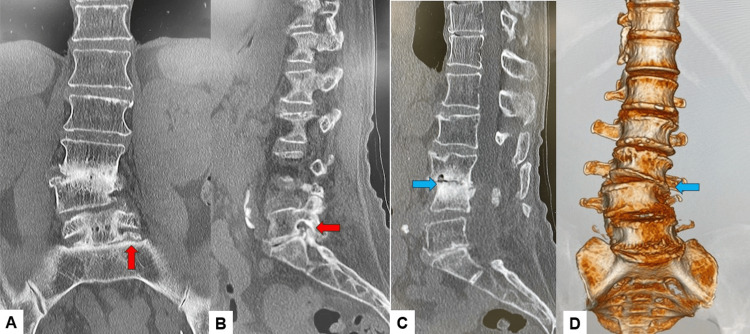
Preoperative CT. A: Coronal reconstructed CT image; B: left parasagittal reconstructed CT image; C: midsagittal reconstructed CT image; D: 3D CT image. Red arrows indicate left foraminal stenosis. Blue arrows indicate L3/4 angulation and osteoarthritic changes.
